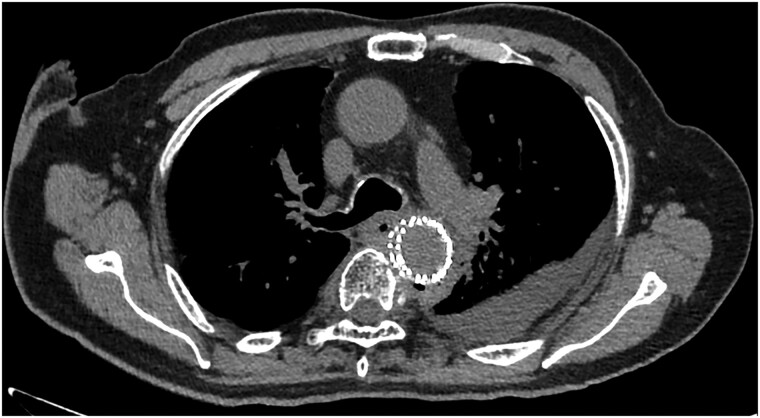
Because postoperative computed tomography revealed the air around the stent graft, the patient was diagnosed with stent graft infection 25 days after thoracic endovascular aortic repair.
